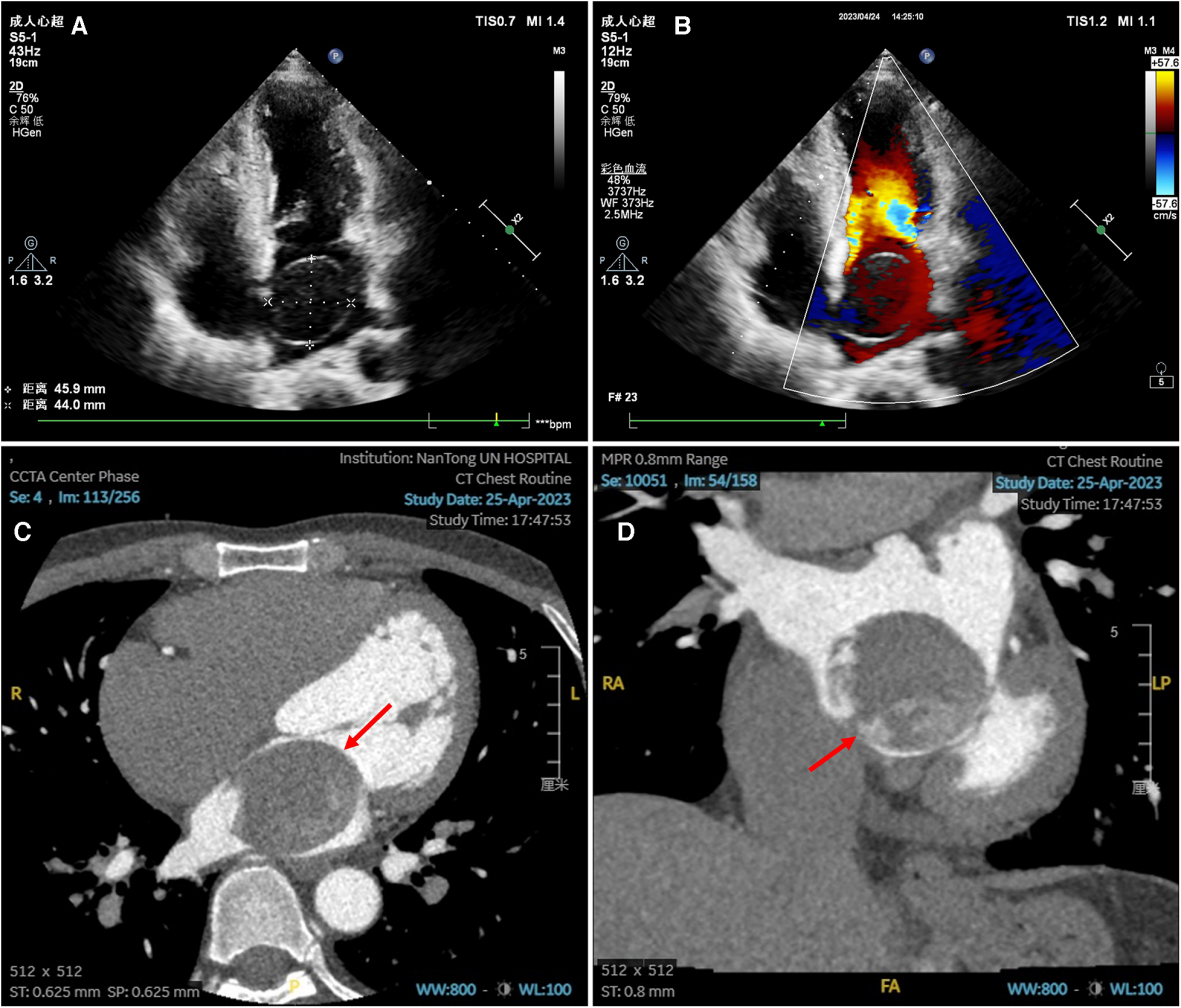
Preoperative echocardiography and enhanced cardiac CT scan images. (A–B) Transthoracic echocardiography showed an echoless tumor measuring 4.6 × 4.4 cm in the left atrium, with clear boundary, a small base attached to the atrial septum, and no blood signal in the tumor. (C–D) Enhanced cardiac CT scan in transverse plane (C) and coronal plane (D) showed a giant tumor in the left atrium with heterogeneous density after enhancement.
